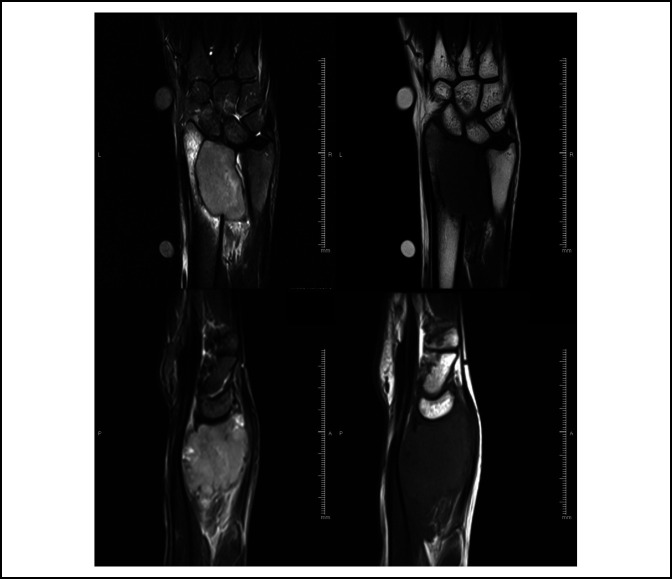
Magnetic resonance imaging demonstrating coronal and sagittal views in T1 and T2 of the distal radius.
